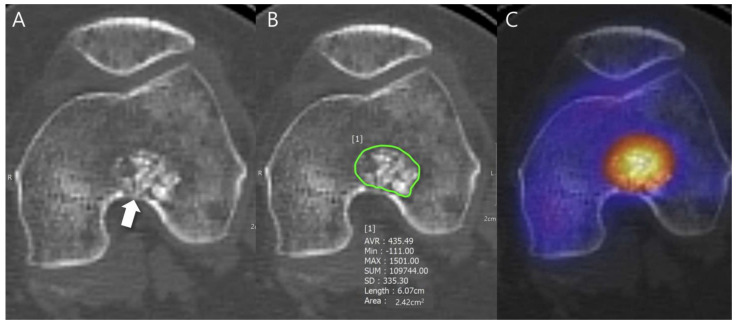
Radiodensity measurement in a patient with ACT in the distal femur. (A) Axial CT image shows a lobulated mass containing chondroid matrix mineralization and focal deep endosteal scalloping ≥ 2/3 of the normal cortical thickness (arrow). (B) Mean (±SD) attenuation of this lesion was measured using the freehand ROI tool (green line) and HU values were found to be 435.49 ± 335.30 [−111 to 1501]. (C) Fused SPECT/CT image shows the radioactive uptake with SUVmax of this lesion which was calculated to be 23.71.
